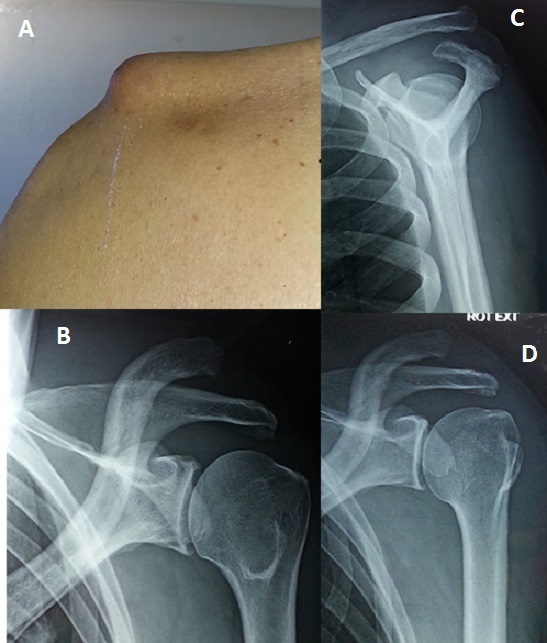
(A) épaule vue du dos: on note que la clavicule remonte en « touche de piano »; (B) radiographie de l’épaule de face: la disjonction acromio-claviculaire est nettement visible; (C) radiographie de l’épaule de profil: disjonction acromio-claviculaire; (D) radiographie de l’épaule en rotation externe montrant la disjonction acromio-claviculaire
